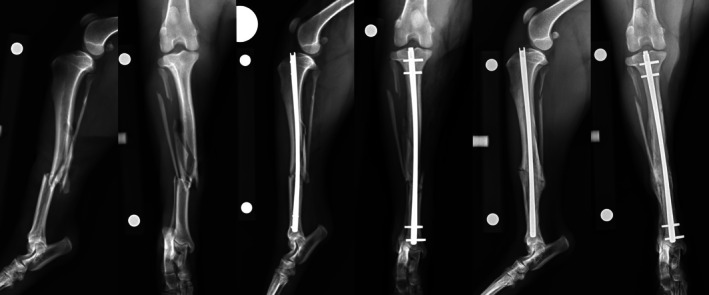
Nail modifications. Preoperative, immediate and 6‐week postoperative radiographs of a 4‐year‐old Beagle with a Gustilo‐Anderson type II open, chronic, comminuted fracture of the mid‐tibial diaphysis. The fracture was stabilized using a 4 × 126 mm I‐Loc AS‐ILN in 2:2 configuration with modification of the tip of the nail to allow deep seating.
